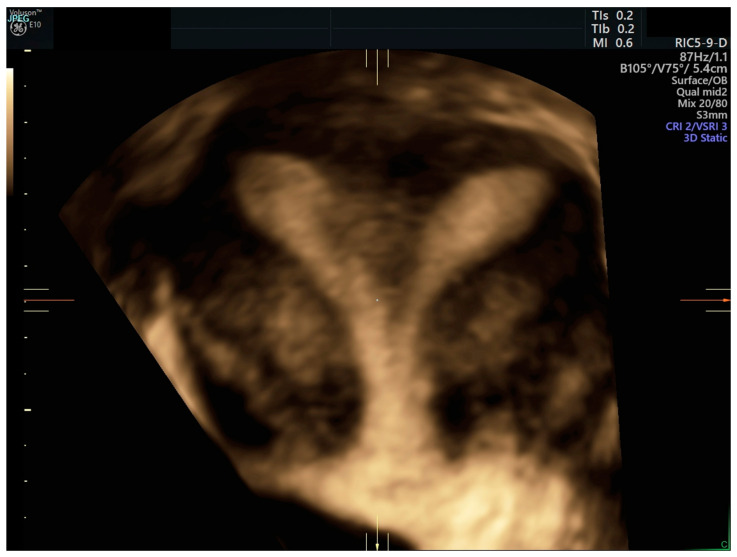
Uterus didelphys showing in 3D ultrasound mode.
